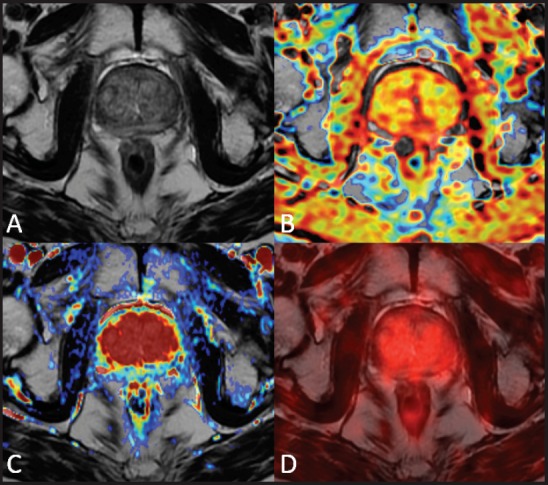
A 75-year-old patient treated with external beam radiation therapy. The T2-weighted axial image (A) shows diffuse low signal intensity of the prostate gland with loss of the normal zonal anatomy. The ADC map obtained from the diffusion-weighted sequence does not show any significant focus of restricted water diffusion (B) The transition zone is homogeneously hypervascular on the perfusion map obtained from the DCE–MRI acquisition (C) The ^18^F–choline–PET/MRI fused axial image (D) does not show any focal accumulation of the tracer in the prostate parenchyma. This case underscores that diffusely reduced signal intensity of the prostate parenchyma after RT with loss of zonal anatomy is not necessarily a sign of local recurrence. Images obtained with Quanta Prostate, Camelot Biomedical Systems s.r.l., Genoa, Italy.
